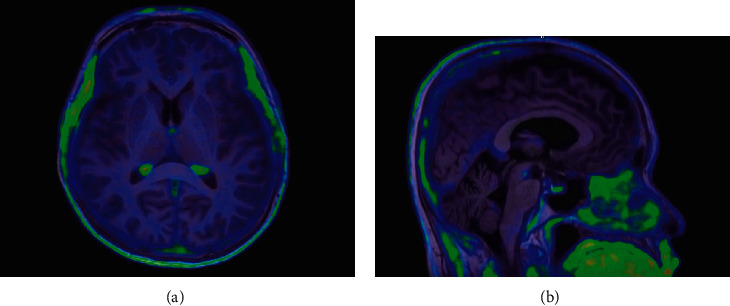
Fusion images between [^18^F]PI-2620 PET and T1-weighted MRI in a CN subject. (a) No uptake of [^18^F]PI-2620 in the bilateral striatum, thalamus, white matter, and whole cortical region. (b) Subtle off-target binding in the midbrain and cingulate gyrus.
